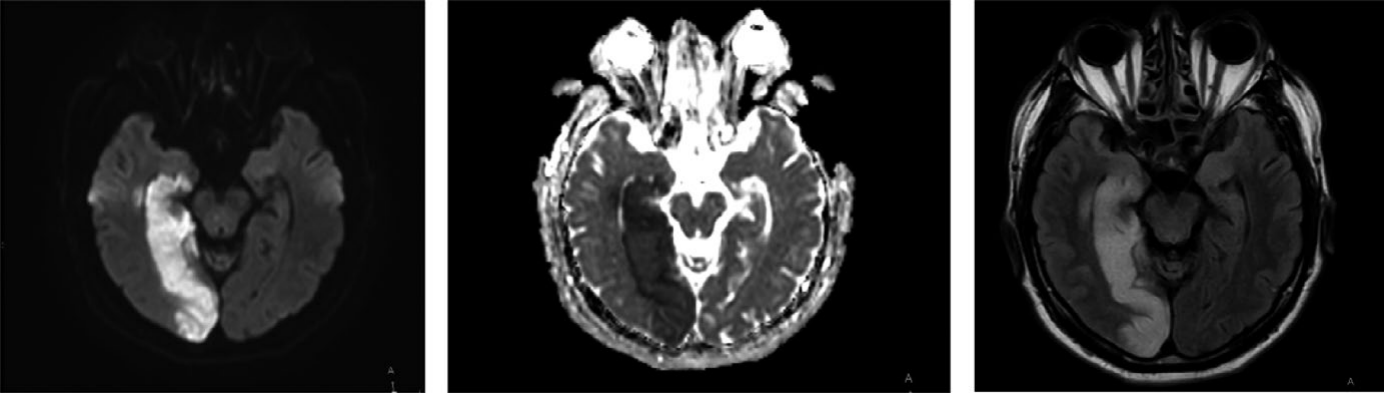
MRI images of the brain in axial projection with an edema and ischemia zone in the right posterior cerebral artery blood supply area
